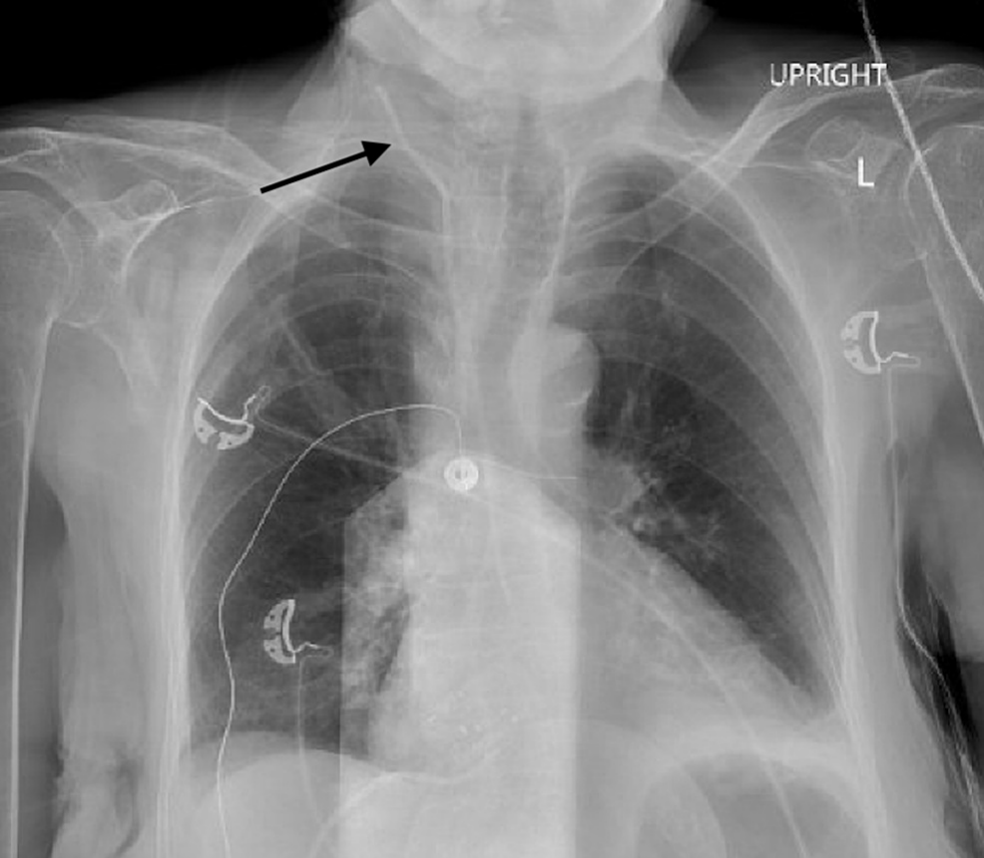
Upright chest X-ray showing the central venous catheter (arrow). L: left.
